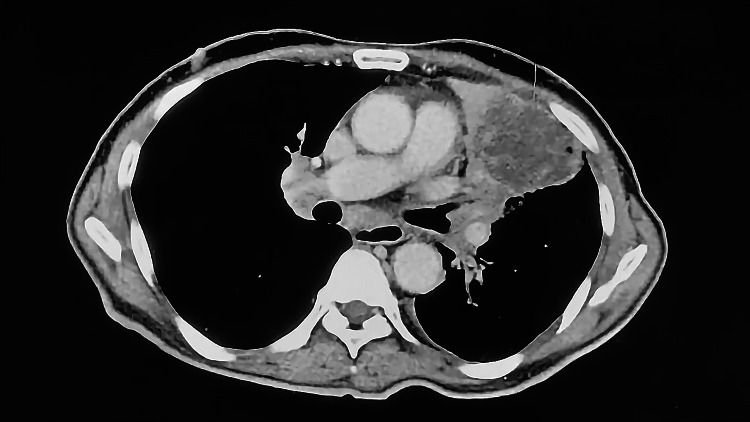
Chest, abdomen, and pelvis CT scan of Patient #1 with contrast (2.9× magnification). CT: computerized tomography
